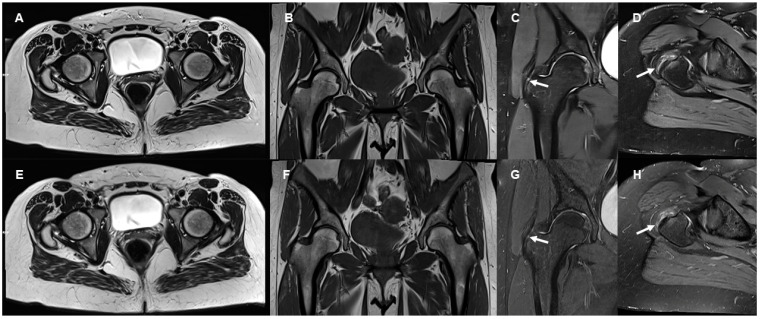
Examples of images comparing TSE_DL_ (A–D) and TSE_S_ (E–H) at 3 Tesla are provided. A 45-year-old female patient with progressive hip pain for 8–12 weeks in the trochanteric region; the MR images provided the diagnosis of abductor tendinopathy. The axial DL-reconstructed T2-weighted TSE image of the pelvis (A) exhibits enhanced sharpness while maintaining a similar overall appearance to the standard reconstruction (E). Similarly, the DL-reconstructed coronal T1-weighted TSE image of the pelvis (B) demonstrates a slight increase in sharpness and reduced artifacts compared with the standard TSE (F). In the DL-reconstructed coronal PD-weighted fat-suppressed TSE image of the right hip, the DL reconstruction exhibits noticeably reduced noise in comparison with the standard reconstructions (G); the small abductor tendon lesion (arrows) is slightly more visible in the DL reconstruction. The abductor tendinopathy with surrounding edema is well demonstrated in both the DL-reconstructed axial oblique PD-weighted image (D) and the corresponding standard reconstruction (H) even though there are some visible banding artifacts in the TSE_DL_ image.
